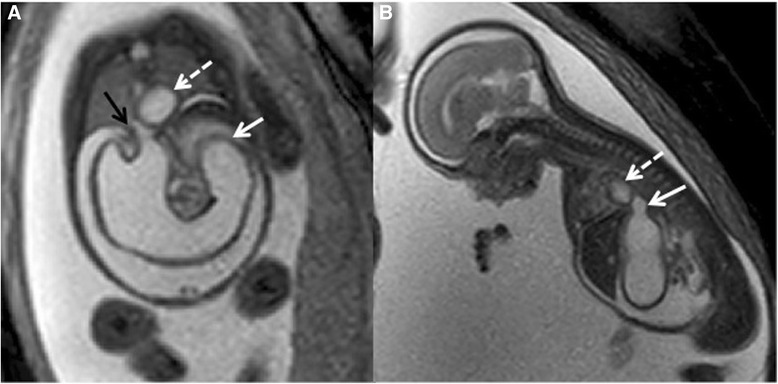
a Coronal T2-weighted magnetic resonance image shows a dilated stomach with cut-off at the second–third duodenal segment (*solid white arrow*), fluid-filled hiatal hernia (*black arrow*), and the dilated distal esophagus (*dashed arrow*) correlating with the cystic structure posterior to the heart seen on the ultrasound. b Sagittal T2-weighted magnetic resonance image shows dilated stomach with fluid dilatation at the gastroesophageal junction consistent with a hiatal hernia (*solid white arrow*). The fluctuating cystic structure posterior to the heart is also seen (*dashed arrow*); it is thought to represent the distal esophagus
